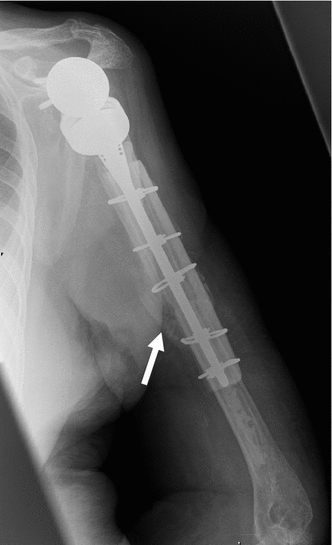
Postoperative plain radiograph of case 1 at 3 months showing well-fixed revision long-stem reverse geometry implant augmented with strut graft. Callus can clearly be seen at the fracture site (*white arrow*)
